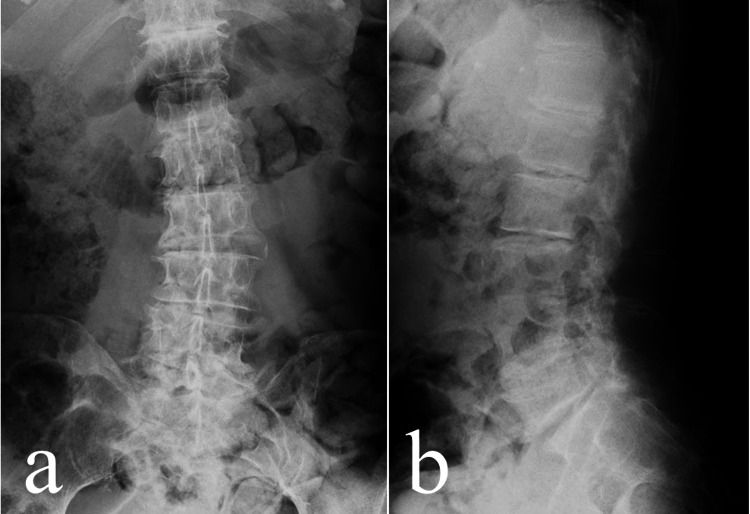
Radiographic image at the first hospital visit a. Anterior-to-posterior view; b. Lateral view Radiographs demonstrated only age-related changes without any abnormal findings
